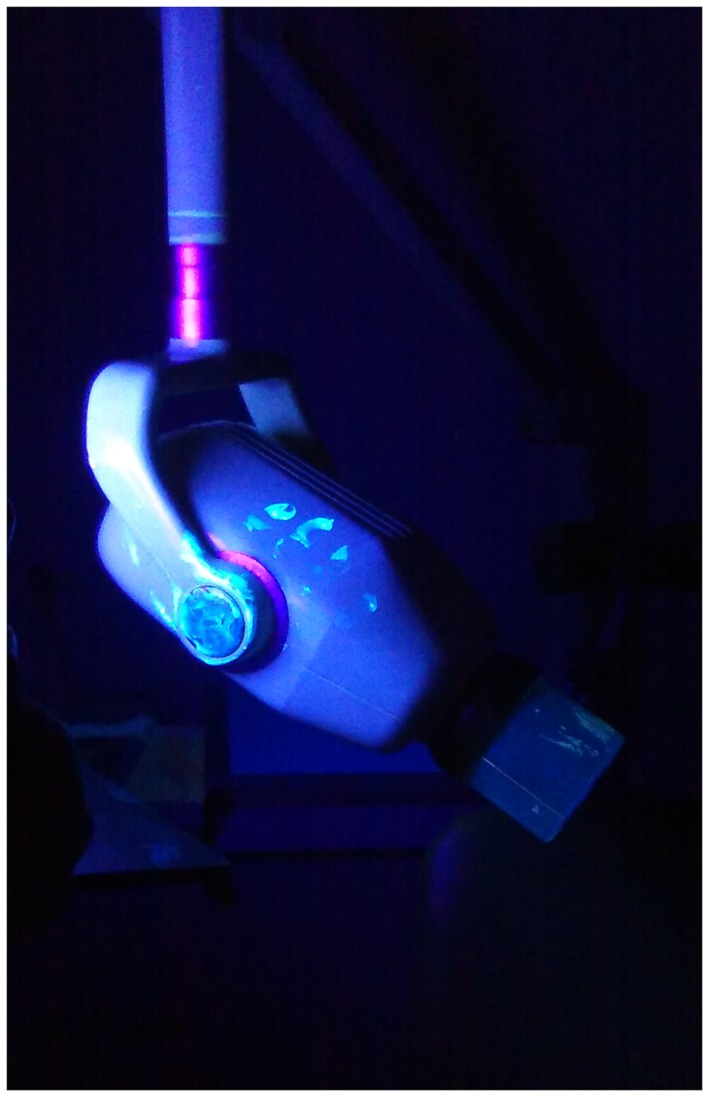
Contamination of radiography equipment, visualized under black light
